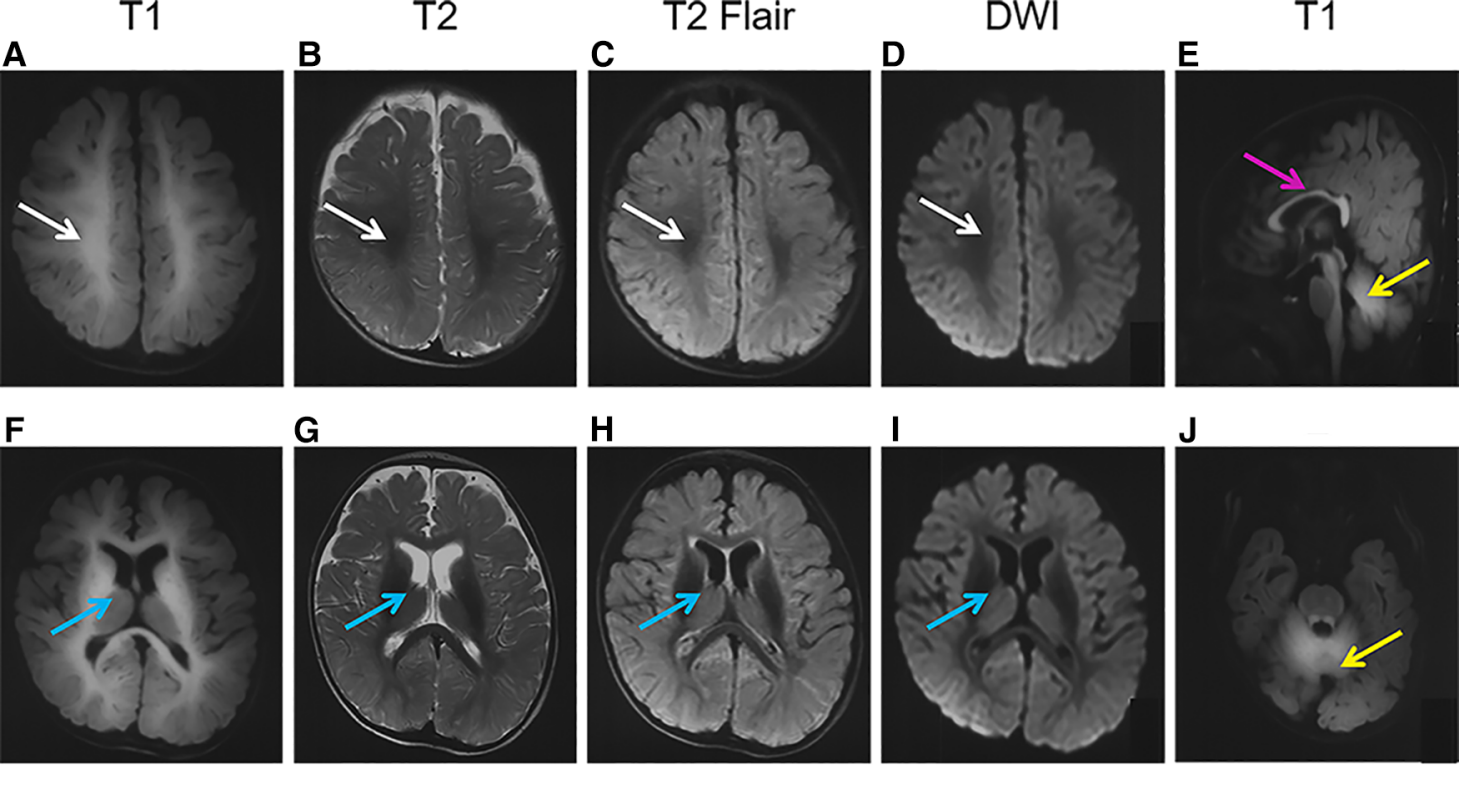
Brain magnetic resonance imaging (MRI) of the patient. The images show a high-T1 (A,F,E,J) and low-T2 (B,G), T2Flair (C,H), and DWI (D,I) signals in the regions of the centrum semiovale (A–D, white arrows), basal ganglia (F-I, blue arrows), corpus callosum (E, pink arrows), and cerebellum (E,J, yellow arrows).
